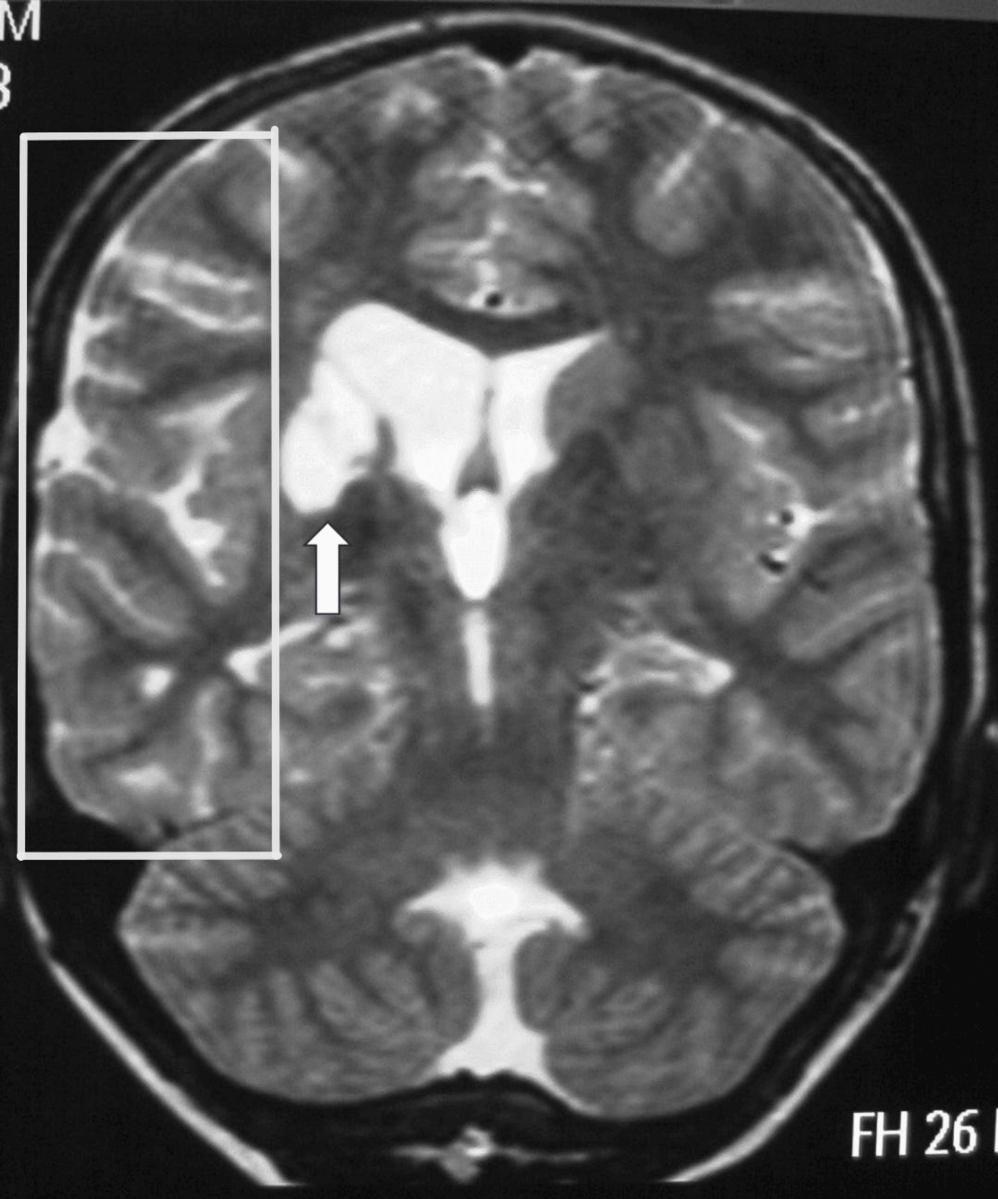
Brain MRI (axial T2-phase) of a female patient with bilateral Moyamoya disease, showing chronic ischemic infarcts and encephalomalacic changes predominantly in the right hemisphere (white outline), corresponding to the ischemic strokes she suffered at the age of 13. The white arrow highlights the periventricular ischemic lesion adjacent to the lateral ventricle. MRI: magnetic resonance imaging
